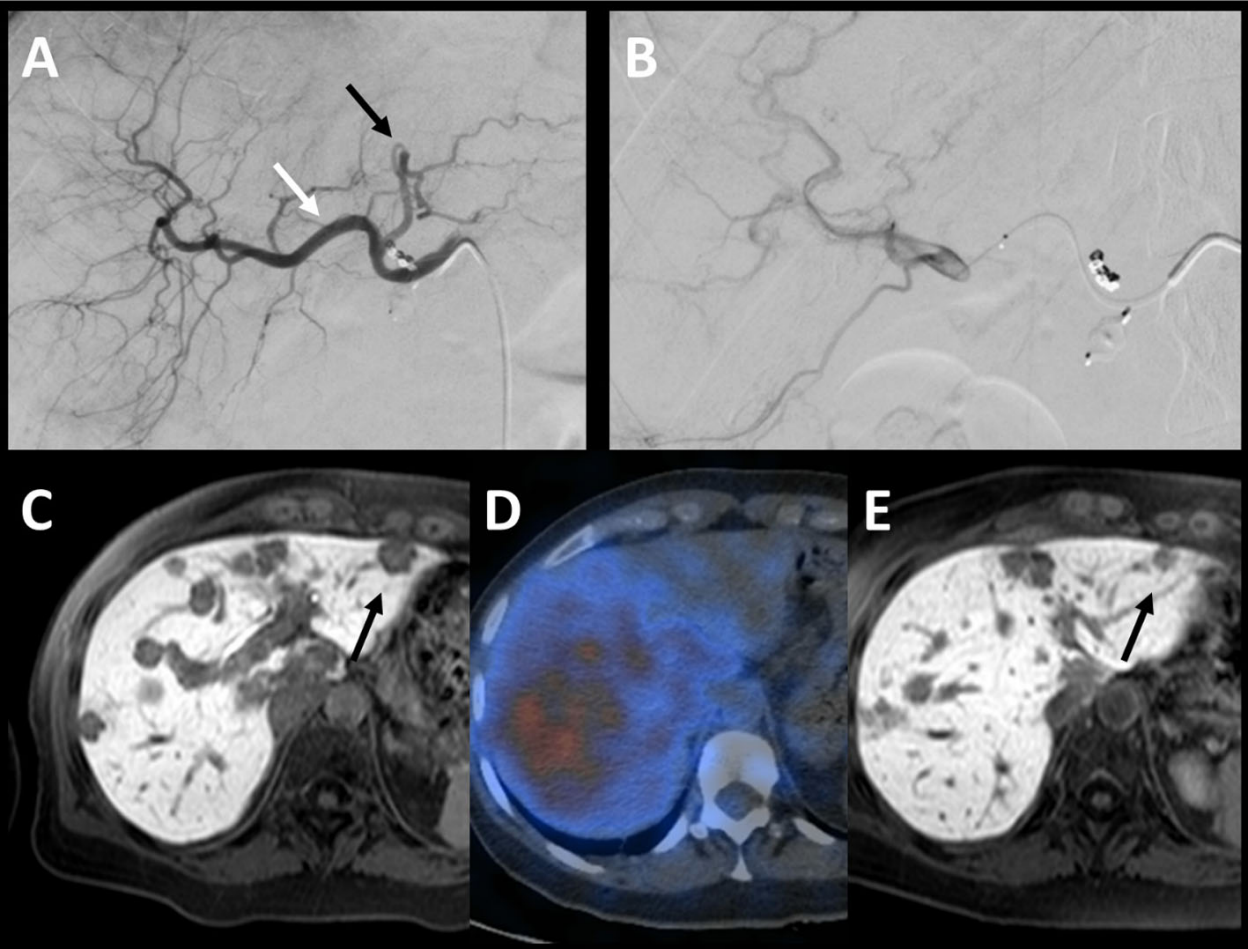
Patient A, breast cancer, A-E, certain abscopal effect: A white arrow demonstrates the injection of Tc-99 m-MAA /the evaluation point of segments 5,6,7,8,1 and partial 4; black arrow shows the evaluation point left and partial segment 4. B application of ^90^Y right (5–8, 1 and partial segment 4). C MRI1: T1w 20 min after administration of primovist—late enhancement phase 1 day prior to RE, black arrow shows metastasis prior to treatment of the right liver lobe. D Bremsstrahlung-SPECT/CT examination: no ^90^Y distribution in the left liver lobe. E MRI2: 42 days after the first RE—black arrow shows untreated metastasis decreased in diameter
